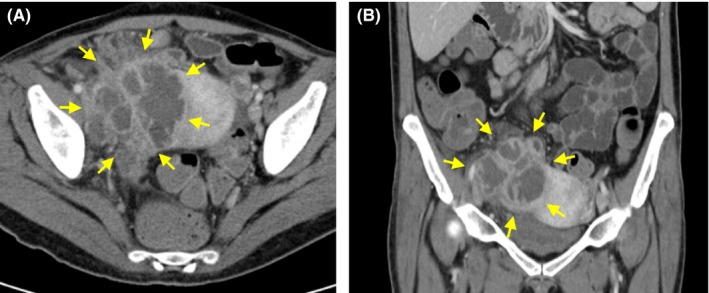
CT scan of abdomen and pelvis on hospital day 3 was obtained due to acute worsening of clinical picture, including development of generalized abdominal tenderness, persistent fevers up to 102° Fahrenheit, and worsening leukocytosis. Imaging findings demonstrated a septated soft tissue collection in the right adnexal region (yellow arrows) that has increased in size compared to imaging on admission. Again, this collection may represent an enlarging appendiceal abscess vs tubo‐ovarian abscess. A, Axial view. B, Coronal view
